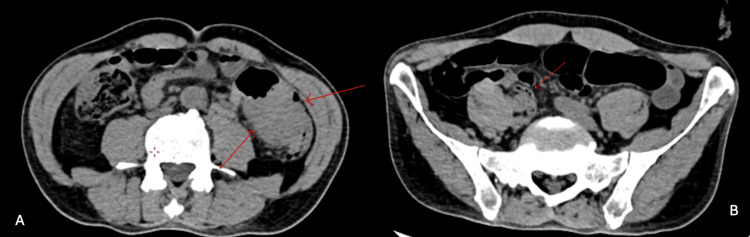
Abdominal CT - Case 1 Case 1. A: Tumor in the descending colon. B: Appendix. The CT reported marked circumferential parietal thickening of the middle/proximal third of the transverse colon, close to the hepatic angle. It didn't report appendicular diverticulosis or diverticulitis, only making the diagnosis of AD after the biopsy was studied.
